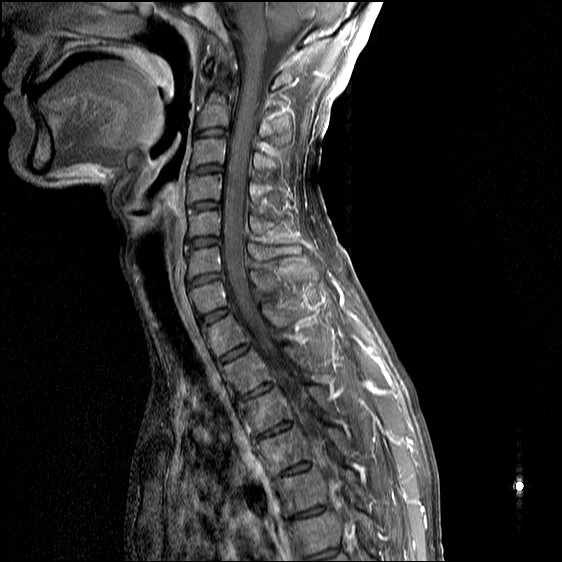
Magnetic resonance imaging scan of the spine from May 2011. The magnetic resonance imaging scan acquired in May 2011 indicated no evidence of osteitis in the cervical spine.
